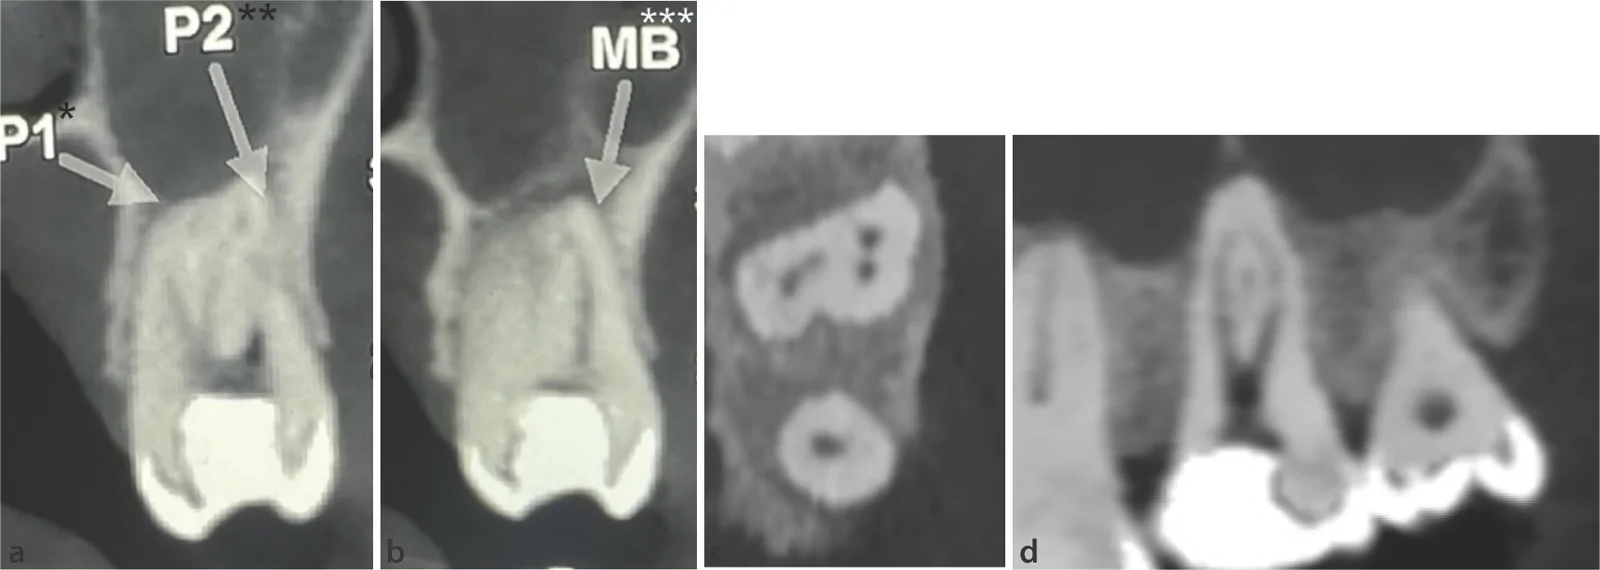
CBCT images a: Coronal view, b: Coronal view, c: Axial view, d: Sagittal view (*P1: palatal canal 1, P2: palatal canal 2, *MB: mesiobuccal)
